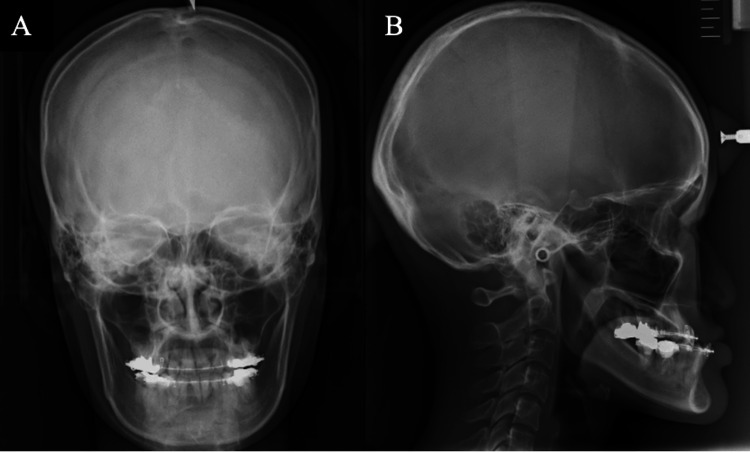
Preoperative radiographs (A) Posteroanterior cephalometric radiographs. (B) Lateral cephalometric radiographs. Based on the cephalometric radiograph taken at the initial examination, the patient was diagnosed with mandibular prognathism.
